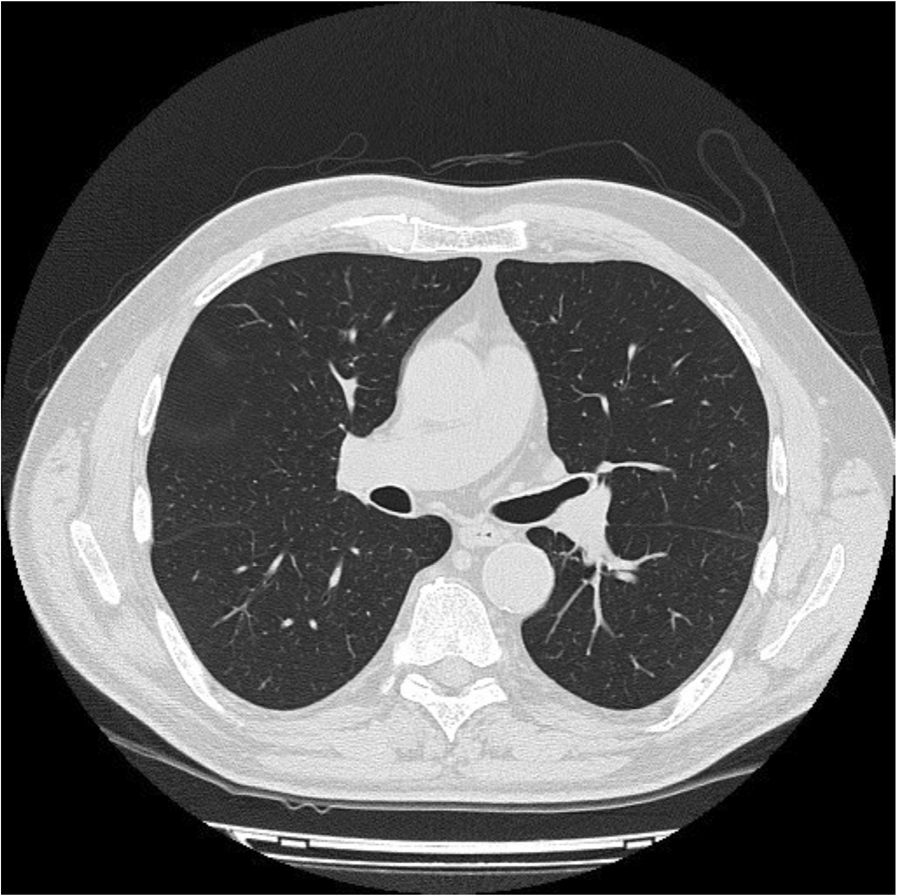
Axial HRCT scan of the chest showing complete radiological pulmonary resolution at 3 years from diagnosis
